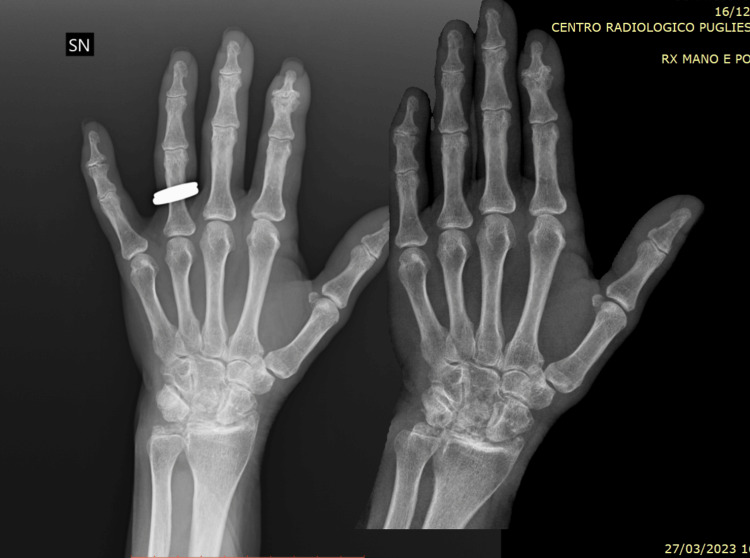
Comparison of two radiographs of the left hand and wrist taken one year apart, demonstrating visible progression of radiographic damage at the wrist, with the development of new erosions and geodes
